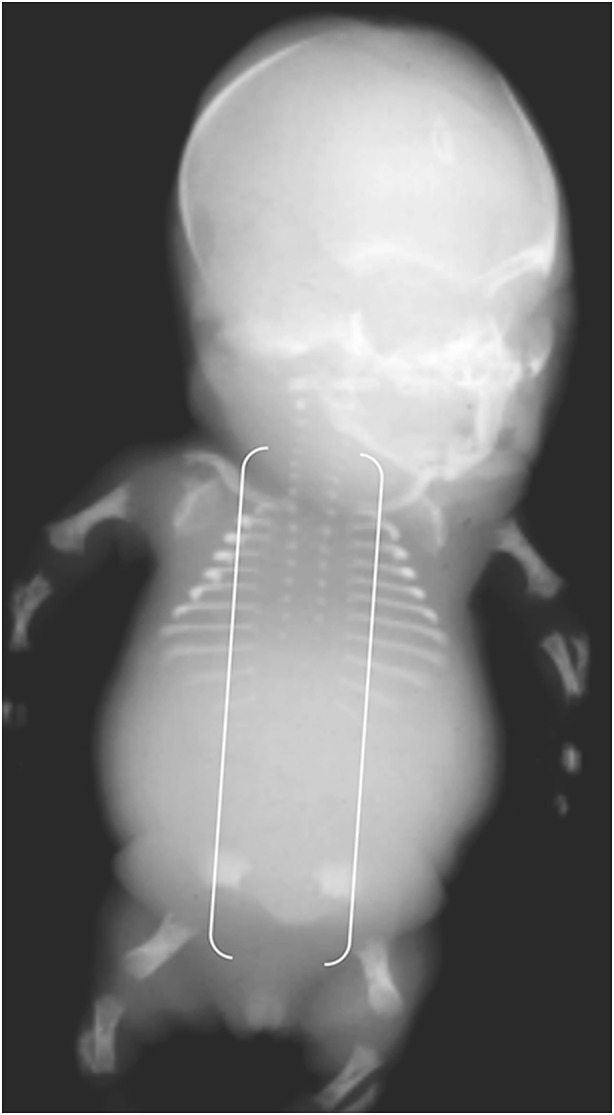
Radiographic features of a male fetus with achondrogenesis type II*.* Anteroposterior radiograph of the least severe form of the disease shows less marked shortening of ribs and long bones, and improved length of long bones and pelvic ossification; however, there is absent ossification of all vertebral bodies and of the lower thoracic, lumbar and sacral pedicles (*brackets*). [Image reproduced with permission from Radcliffe Publishing [38], page 65, case 4]
